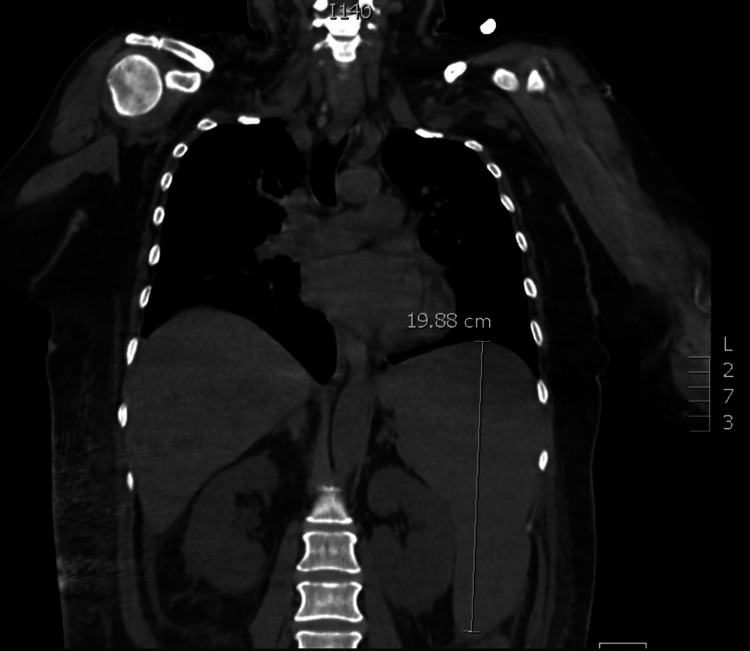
CT of the chest/abdomen showing severe splenomegaly
